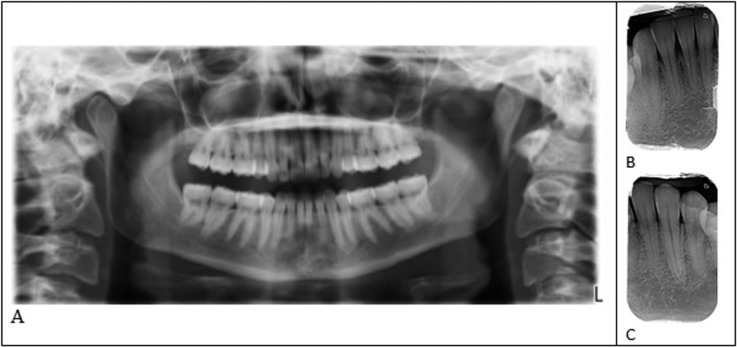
A. Panoramic radiography. B. Retroalveolar radiography teeth 32-43. C. Retroalveolar radiography, teeth 32-34.
